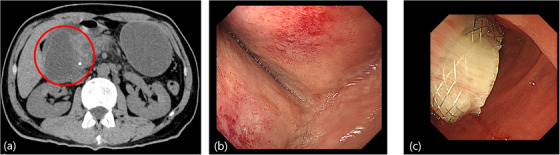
Preoperative case of pancreatic head cancer with duodenal bulb route of lumen‐apposing metal stents (LAMS) placement. (a) The patient was admitted to our hospital with vomiting and anorexia. The patient had undergone insertion of a plastic stent into the common bile duct because of obstructive cholangitis caused by cancer of the pancreatic head. Contrast‐enhanced computed tomography revealed obstructive pancreatitis due to the pancreatic head cancer and pancreatic fluid collections (red circle) on the tail side of the pancreatic head cancer. (b) Upper endoscopy revealed extrinsic compression by pancreatic fluid collections of the pancreatic head in the duodenal bulb. The endoscope encountered difficulty passing to the second part of the duodenum, revealing a passage obstruction. (c) In this case, due to the preoperative state of pancreatic head cancer, the site of LAMS placement was selected using the duodenal bulb route, which is within the scope of subtotal stomach‐preserving pancreaticoduodenectomy.
